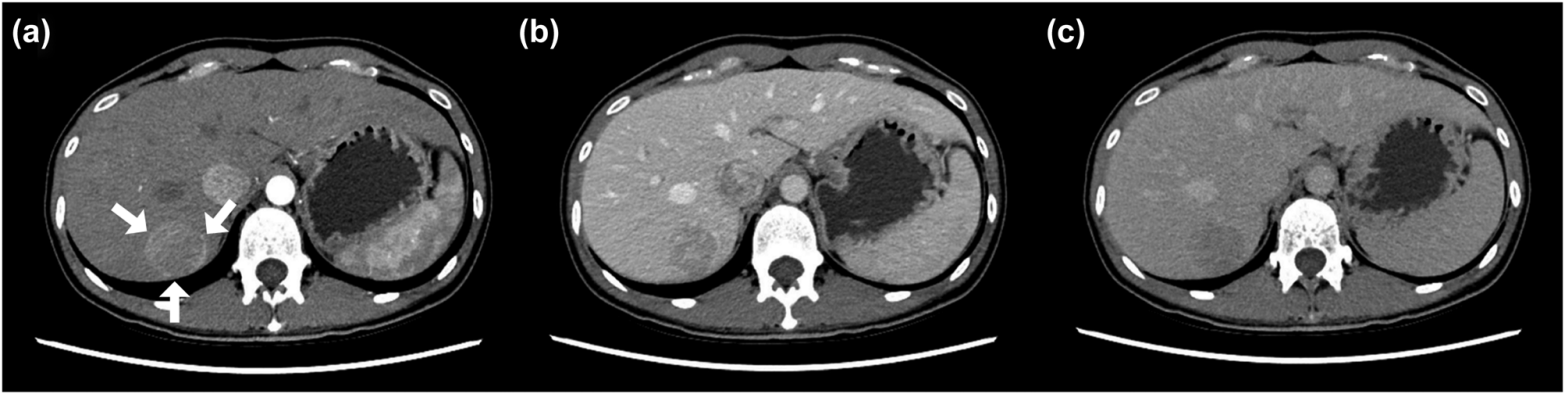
Contrast-enhanced computed tomographic (CT) imaging of a primary hepatic perivascular epithelioid cell tumor in the arterial (a, 25–30 s), portal (b, 60 s), and equilibrium (c, 180 s) phases. A heterogeneous enhancement can be seen during the arterial phase, while the contrast agent was washed out during the portal and equilibrium phases (arrows).
